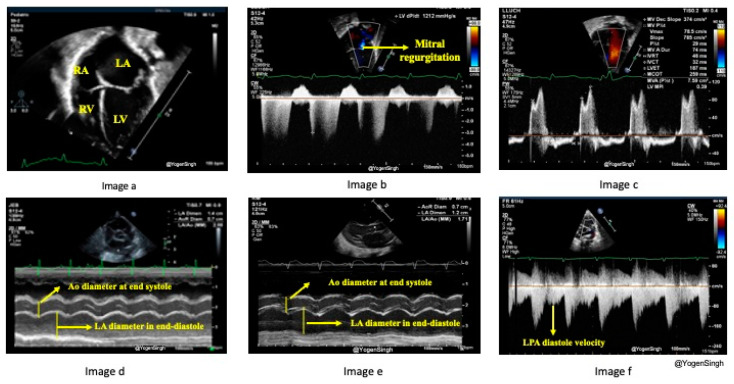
Echocardiography assessment of pulmonary over-circulation. (a) shows the left heart is larger than the right heart due to volume overload in the apical four-chamber view. (b) shows the mitral regurgitation on color flow mapping from the apical four-chamber view. (c) shows E/A ratio inversion across the mitral valve inflow. (d) shows the increased LA to Aorta (LA/Ao) ratio in the parasternal short-axis view on the M-mode. (e) shows the increased LA to Aorta (LA/Ao) ratio on the M-mode in the parasternal long-axis view. (f) shows an increased diastolic velocity in the left pulmonary artery (LPA). *LA—left atrium, LV—left ventricle, RA—right atrium, RV—right ventricle, Ao—aortic valve, LPA—left pulmonary artery*.
